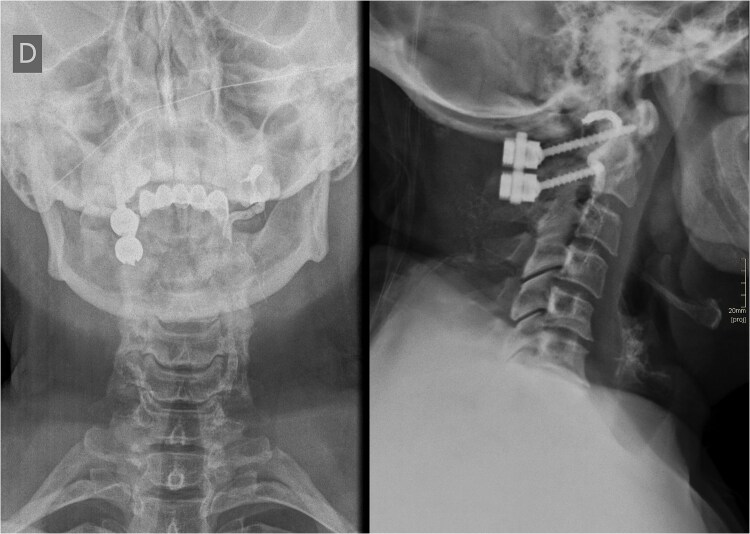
Postoperative X-ray of the cervical spine showing contralateral unilateral C1-C2 fixation and the left VA opacified with a radiopaque contrast agent.
